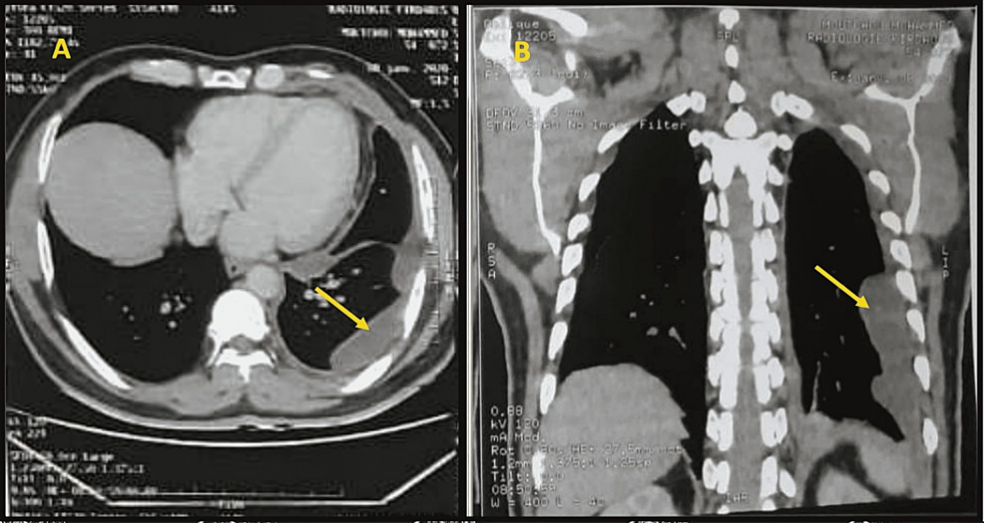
Axial (A) and coronal (B) view of CT chest with contrast showing left nodular thickening of the pleura (yellow arrows)
